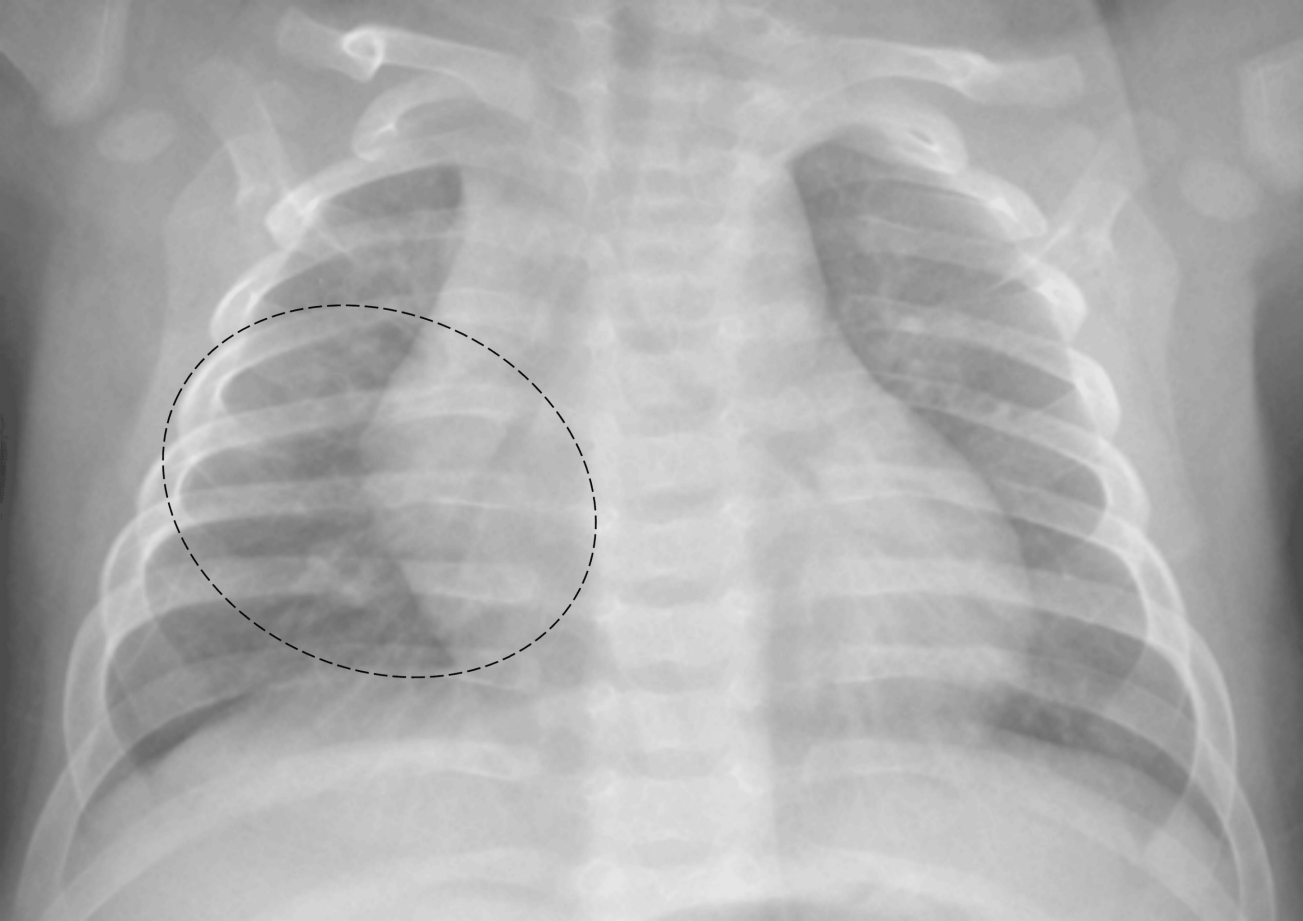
Chest radiograph at two months Anteroposterior chest radiograph at a two-month follow-up, showing a marked improvement (circle)
